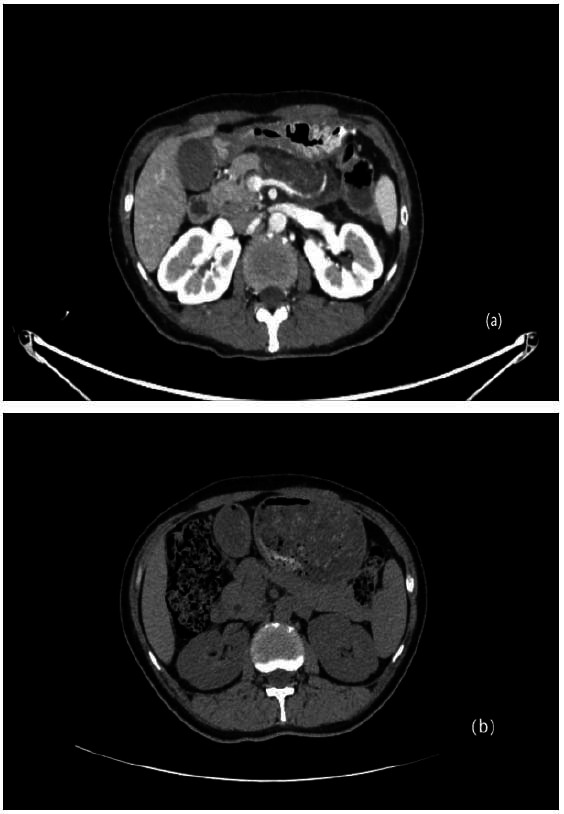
CT scan of the patient’s abdomen (patient identifiers removed). (a) CT scan indicated an AP with a full and plump shape of the pancreatic head on the first day of the HTG-AP. (b) CT scan indicated a normal pancreas after a month of the HTG-AP.
